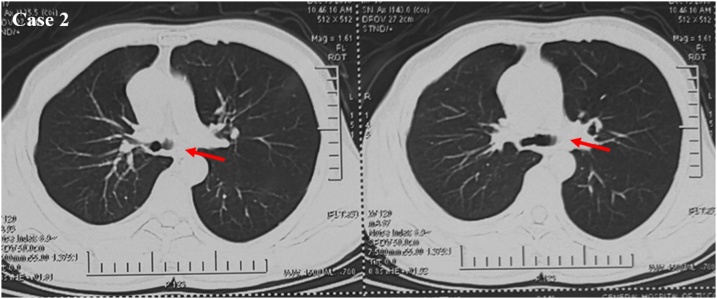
Chest CT in case 2. Tumor infiltrates the middle part of the carina closed to the left main bronchus (see the arrow).
